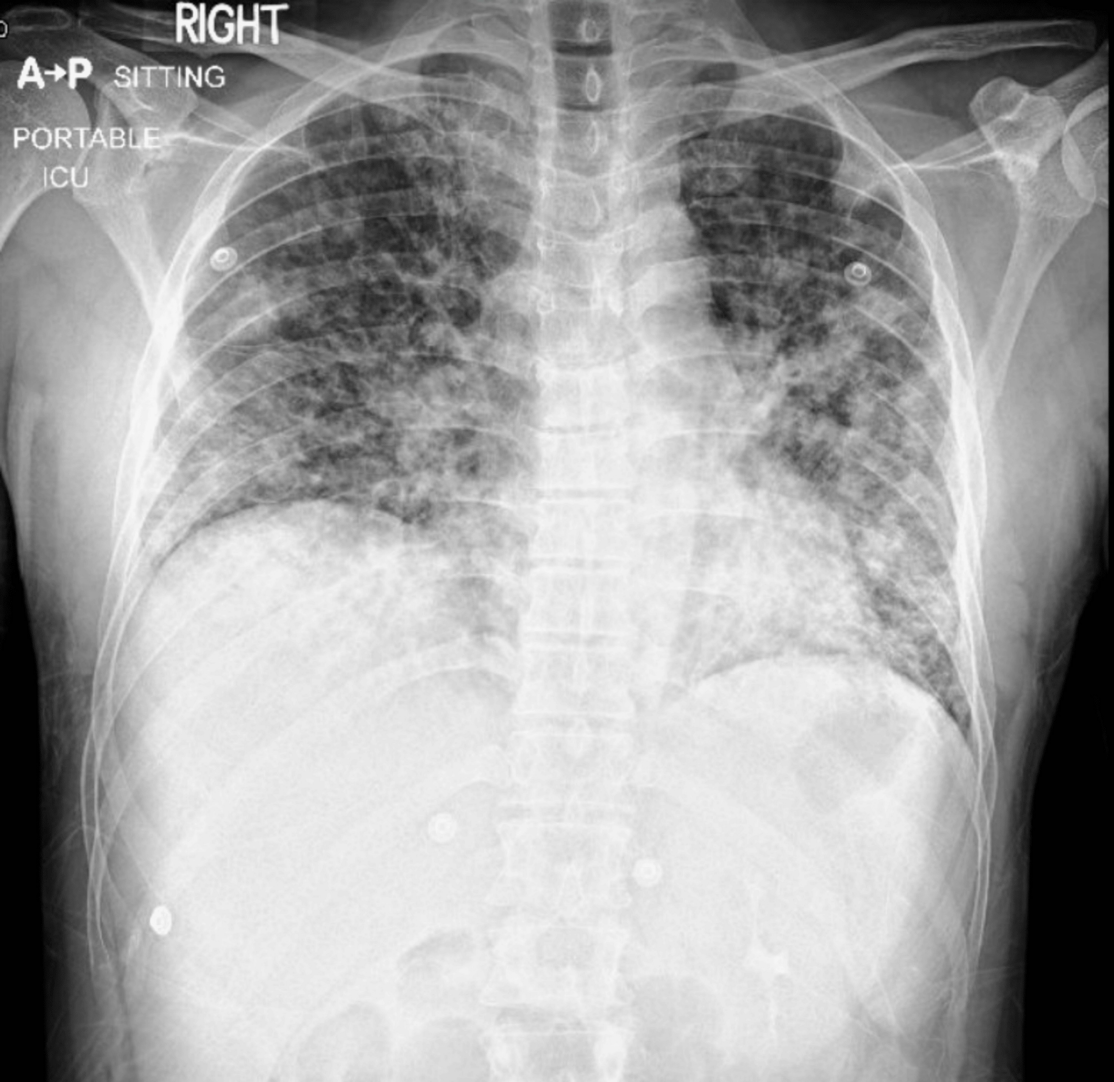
Chest X-ray showing bilateral lung infiltrates
